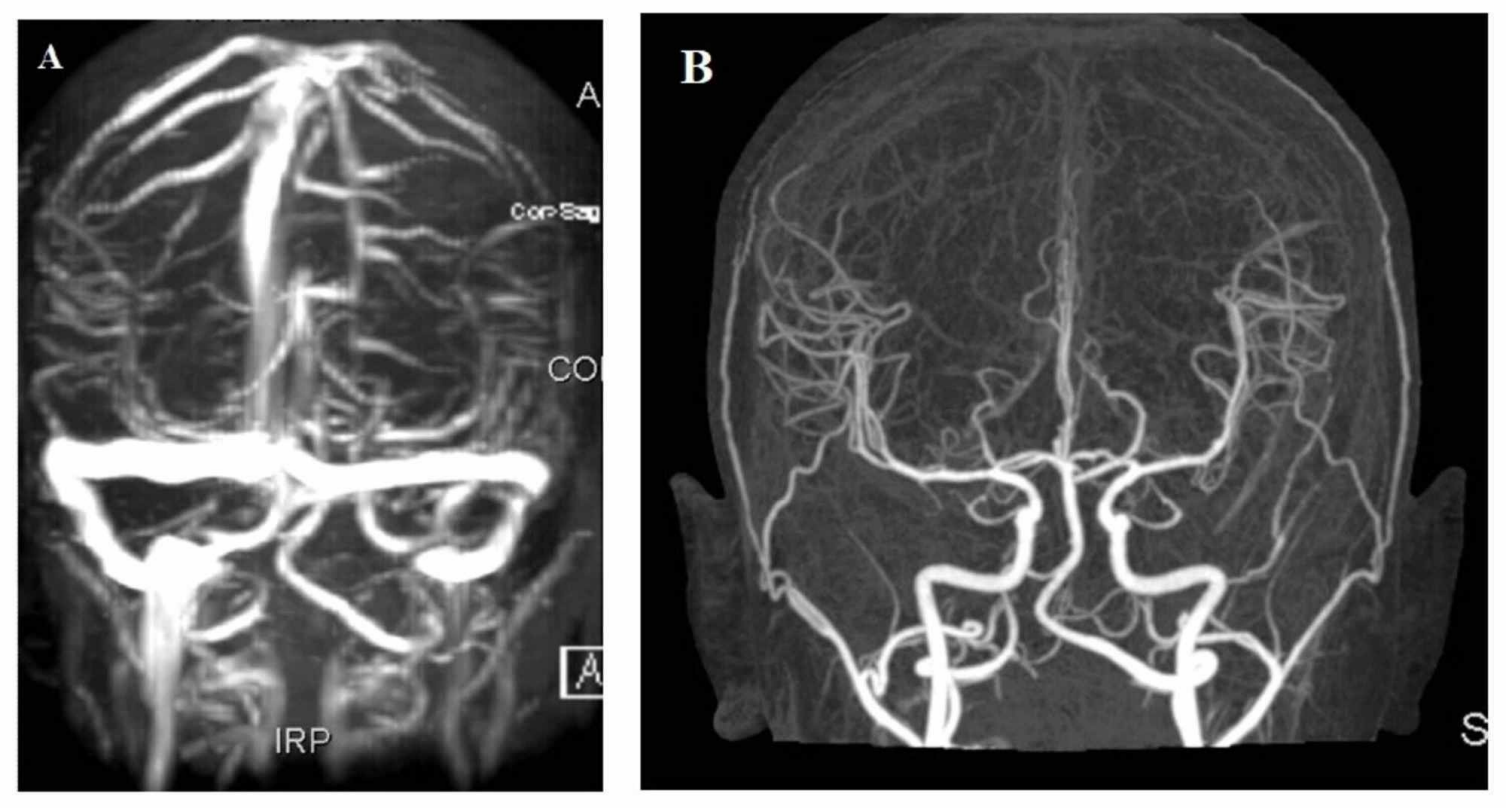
(A) Magnetic resonance venography with gadolinium contrast showed no evidence for dural venous sinus thrombosis, (B) CT cerebral angiography showed arteries are patent with no evidence for any aneurysms or arteriovenous malformation
